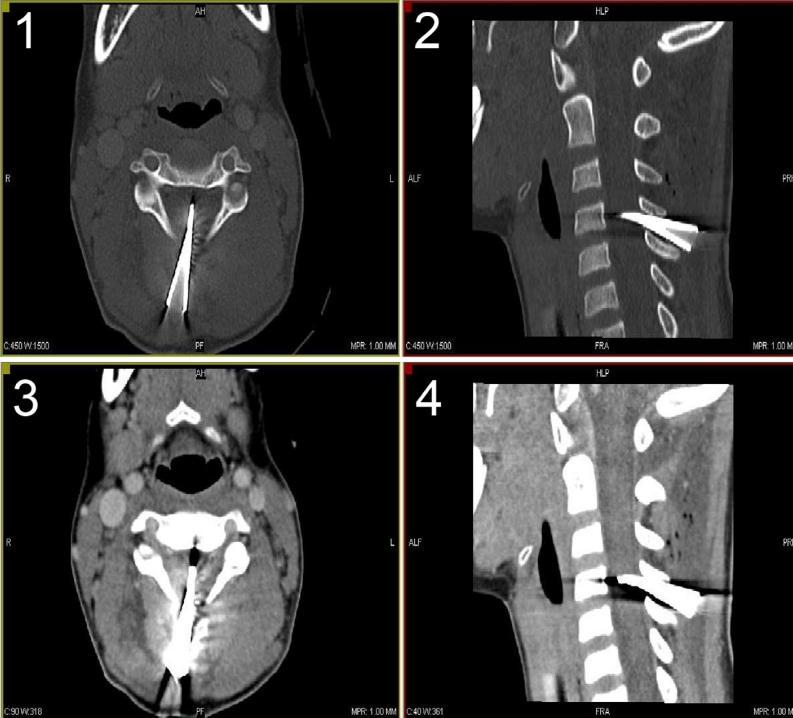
CT scans showing arrowhead stuck within the vertebral canal. *1*,*2* bone frame (axial and sagittal aspect); *3*,*4* nervous tissue frame (axial and sagittal aspect)
